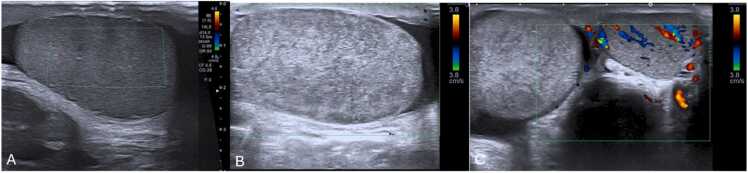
(A) Scrotal sagittal color-doppler US of a 12 year-old male with testicular torsion, obtained less than 6 h after symptom onset. Notice that although there is loss of vascularity, the testis has preserved echogenicity and homogenous echotexture, indicating the viability of the tissues. Compare with the sagittal and transverse images (B and C) of a testicular color-doppler US of another 17 year-old-male, obtained 12 h after symptom onset, showing an enlarged right testicle (volume of 24 cc vs. 13 cc of the left) with loss of vascular flow, and a heterogeneous parenchyma, containing areas of hyper- and-hypo echogenicity related to edema and swelling. Notice the difference in size, echotexture and vascularity of the right in comparison with the left testicle.
